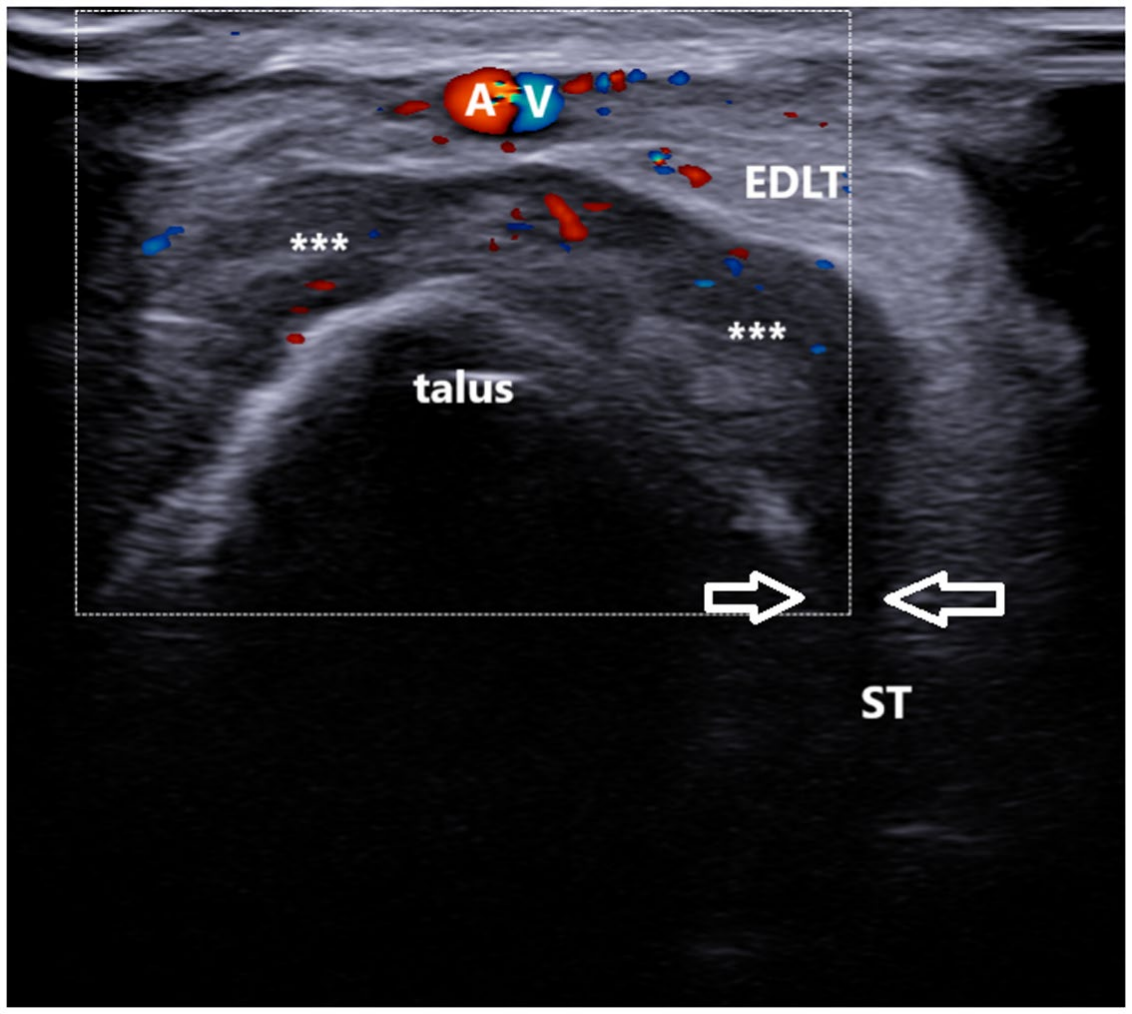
A 37-year-old patient with gout. Ultrasound image showing unilocular, power Doppler-positive GB with the transducer positioned in the short axis of the foot (left side is medial). The transverse ultrasound image of the dorsolateral ankle reveals a hypoechoic to heteroechoic unilocular fluid collection (*) between the extensor digitorum longus tendon (EDLT) and the talus, extending from the sinus tarsi (between arrows). ST, sinus tarsi; A and V, dorsalis pedis artery and vein; and EDLT, extensor digitorum longus tendon.
